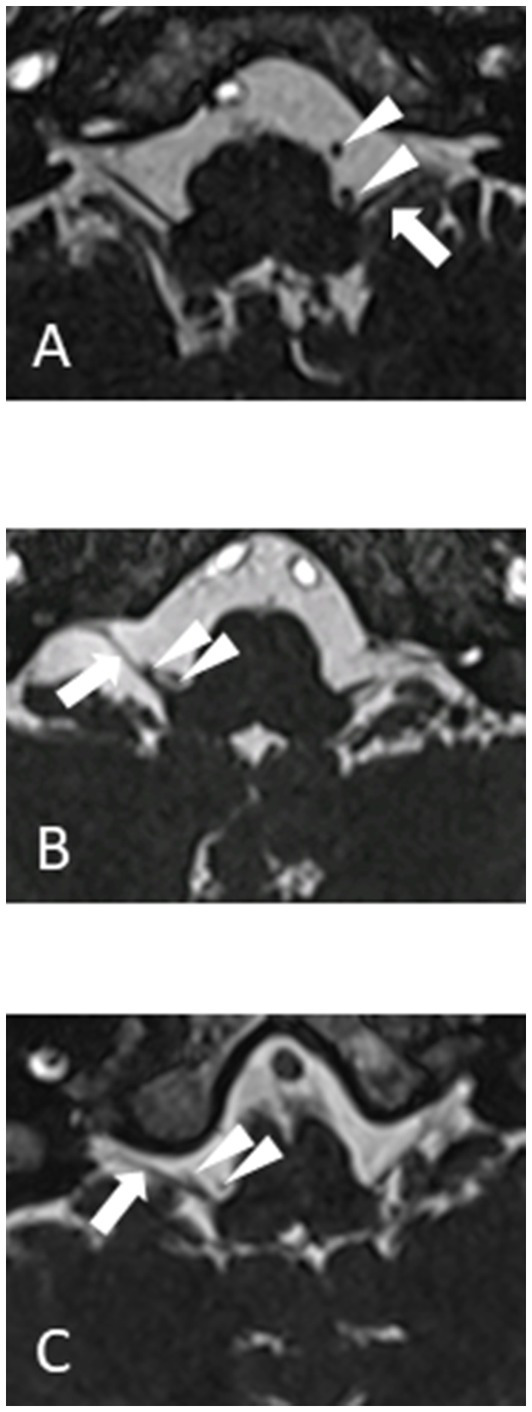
1.5 T MRI CISS sequences of three different patients (A, B and C) showing vagus nerve (arrow) and loop of PICA (arrowheads).
